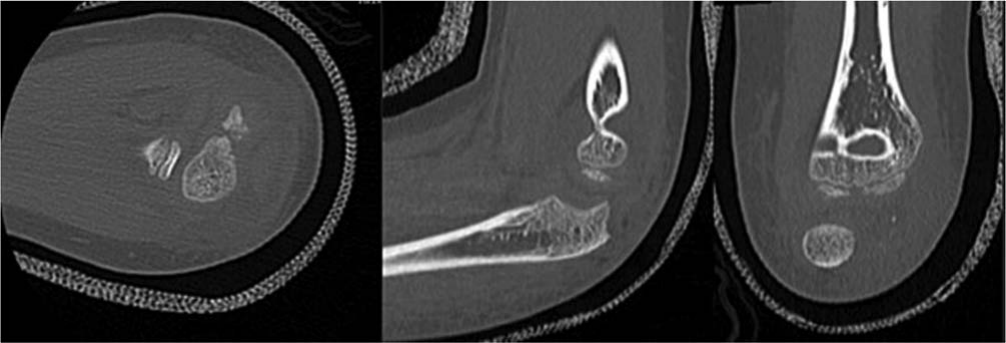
CT scan demonstrating radial neck angulation, radiocapitellar subluxation, and linear calcification near the trochlear notch.
